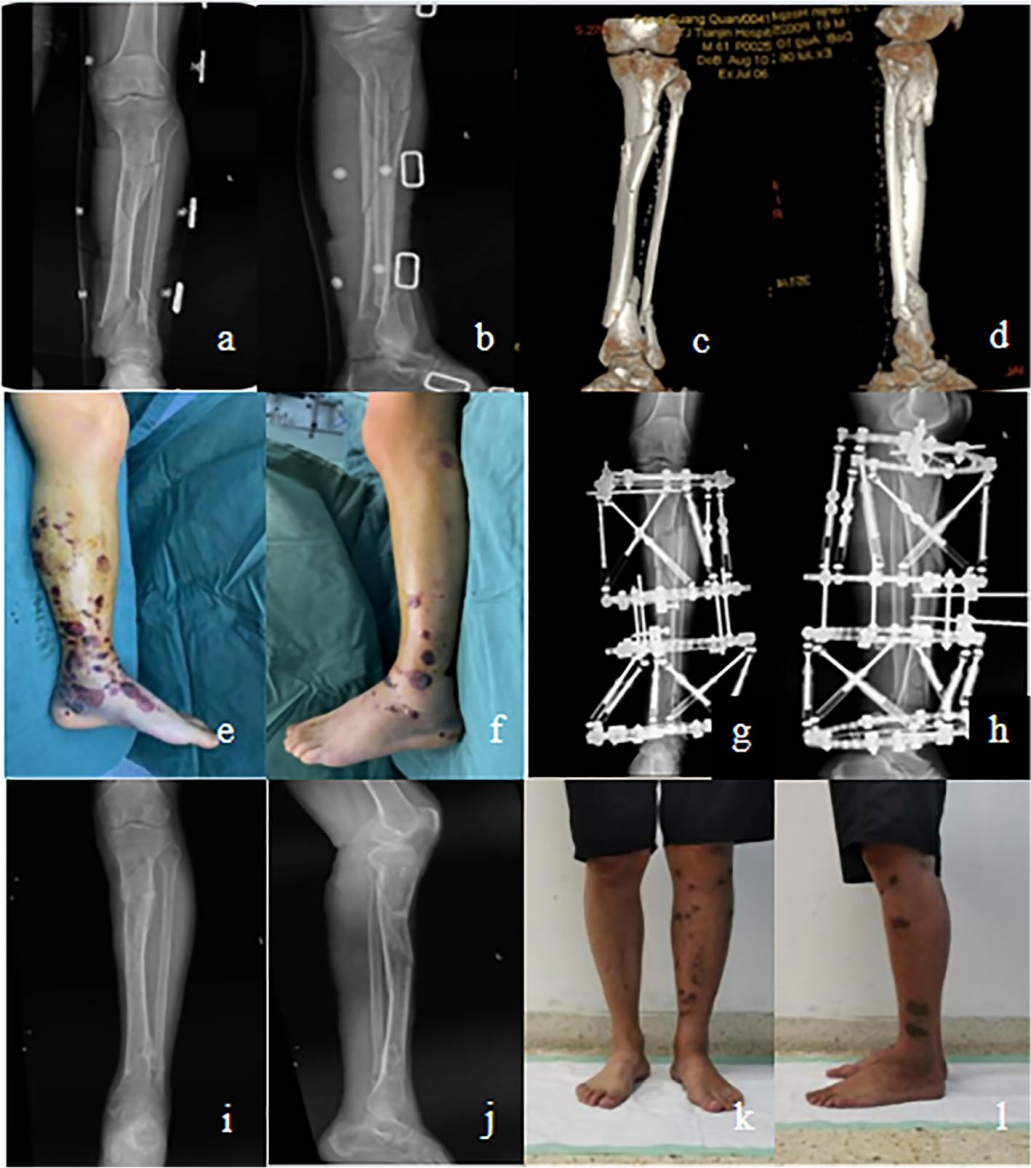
Images of treating a 38-year-old male with STFs due to high falling by using HEF. a, b X-ray images of injured STFs preoperatively. c, d Computer tomography images of injured STFs preoperatively. e, f Appearance images show poor local soft tissue conditions in the left lower leg preoperatively. g, h X-ray images within one week postoperatively. i, j X-ray images show good fracture healing, one week after removal of HEF. k, l Clinical follow-up appearance images obtained 1 month after HEF removal
